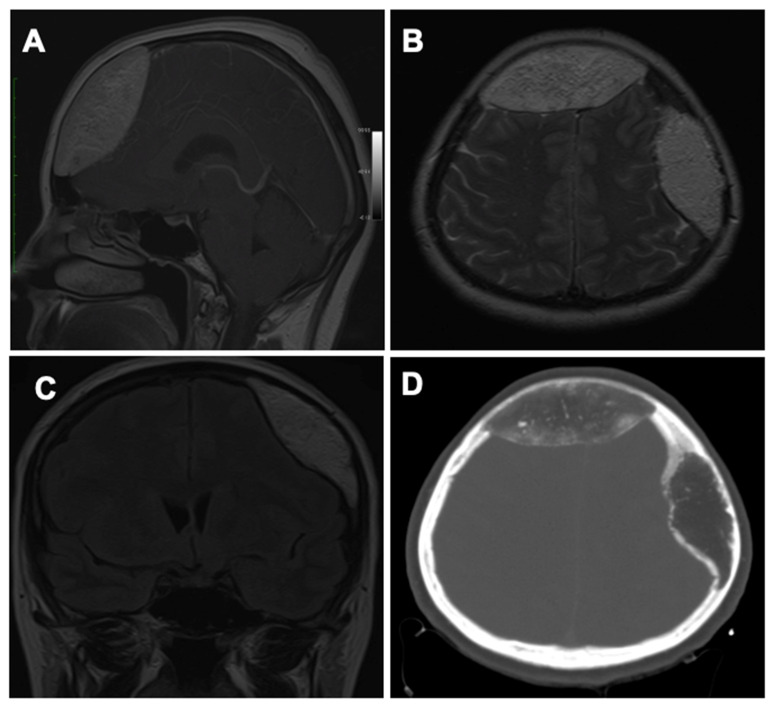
Preoperative neuroimaging: (A) Sagittal MRI scan showing the frontal lesion. (B) Axial MRI scan showing both the frontal and the parietal lesions. (C) Coronal MRI scan showing the parietal lesion. (D) Axial CT scan highlighting the hypodense aspect of frontal and parietal osseous lesions.
